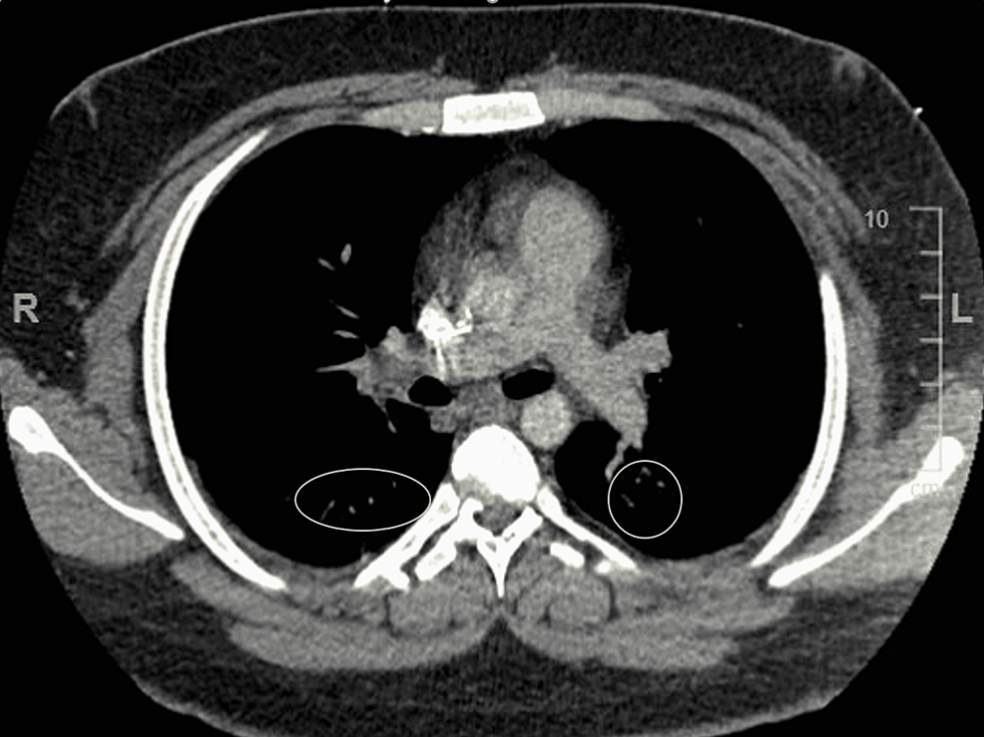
Chest CT angiography showing no acute pulmonary embolism within the central pulmonary arteries Ill-defined ground-glass opacities are shown within the lung bases bilaterally, indicated within the circles.
